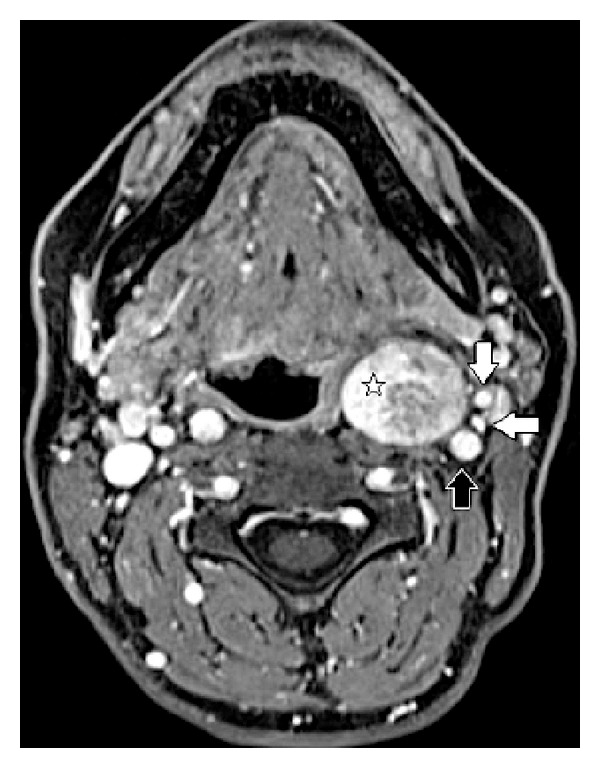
Patient 2, T1-weighted enhanced MRI scan: ovoid mass occupying the left retrostyloid compartment, 3 × 4 cm in size (white star). The internal carotid artery (black arrow), the internal jugular vein (horizontal white arrow), and the external carotid artery (vertical white arrow) are all displaced laterally.
